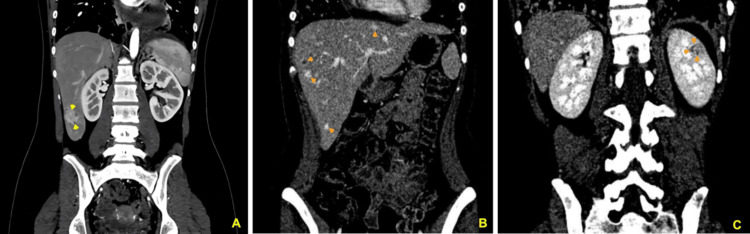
Abdominal CT. (Panel A) A solitary lesion (3.0 × 3.0 cm) (yellow arrowheads) was found in the liver on the initial visit. It had irregular border, hyperenhancement features after contrast administration. (Panels B and C) Follow-up CT after six months of chemotherapy demonstrated multiple nodular lesions (examples include orange arrowheads in Panel B) in the liver and on mass in the left kidney (Panel C, orange arrowheads). CT, computed tomography
